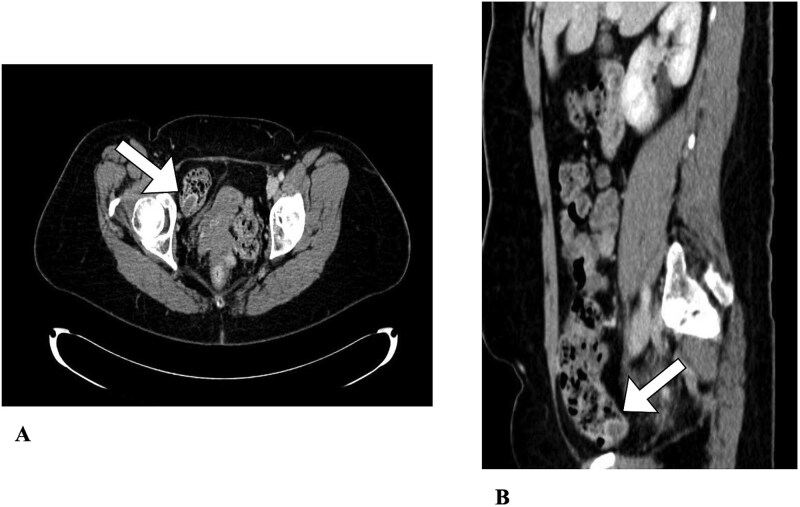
Abdominal CT. An appendiceal cecal intussusception is present (arrows) with the distally located appendix invaginating into the proximally located cecum. (A) Axial view. (B) Sagittal view.
